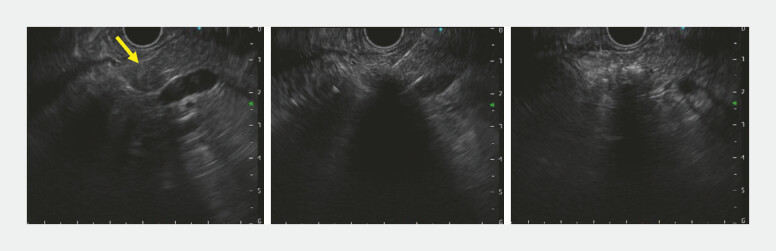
(Left) EUS showed a nearly round pancreatic tumor (arrow). (Middle) EUS-guided ethanol ablation was performed. (Right) The tumor showed uniform hyperechoic changes after EUS-guided ethanol ablation. EUS, endoscopic ultrasound.
